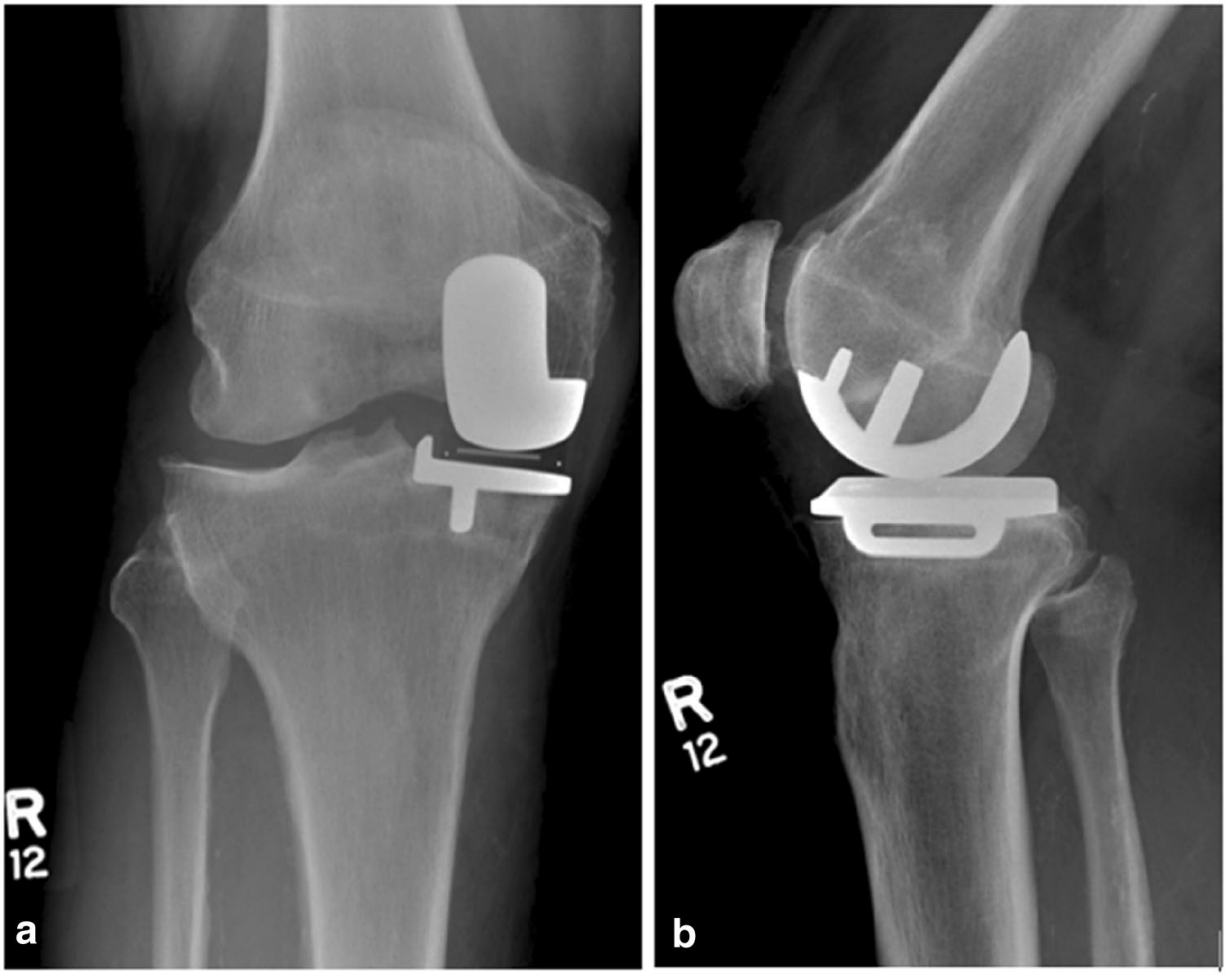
X-Ray of same patient as Fig. 2, showing cementless medial UKR in situ
